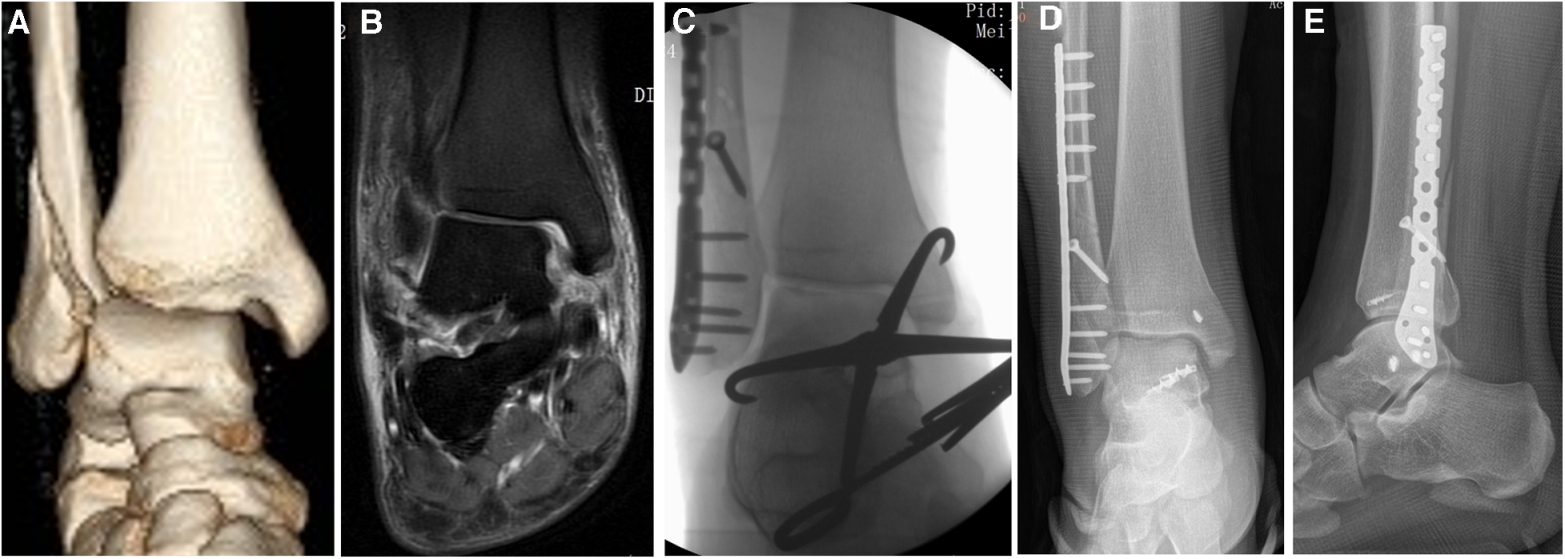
A case of repairment for deep deltoid ligament rupture near the medial malleolar attachment by using suture anchors into the talus combined with transosseous suture in the medial malleolar. (A) Preoperative 3D reconstruction image showed increased medial malleolar space and ankle fracture. (B) Preoperative coronal MRI showed that the ruptured deltoid ligament. Combined with intraoperative exploration, the deltoid ligament was ruptured near the medial malleolar attachment. (C) Intraoperative anterior–posterior x-ray image showed the fixation of the talus—medial ankle relationship with reduction forceps. (D) Postoperative anterior–posterior x-ray image. The deep deltoid ligament was repaired by two suture anchors inserted at the talus. The superficial deltoid ligament was repaired by one suture anchor inserted at the medial malleolar. (E) Postoperative lateral x-ray image.
